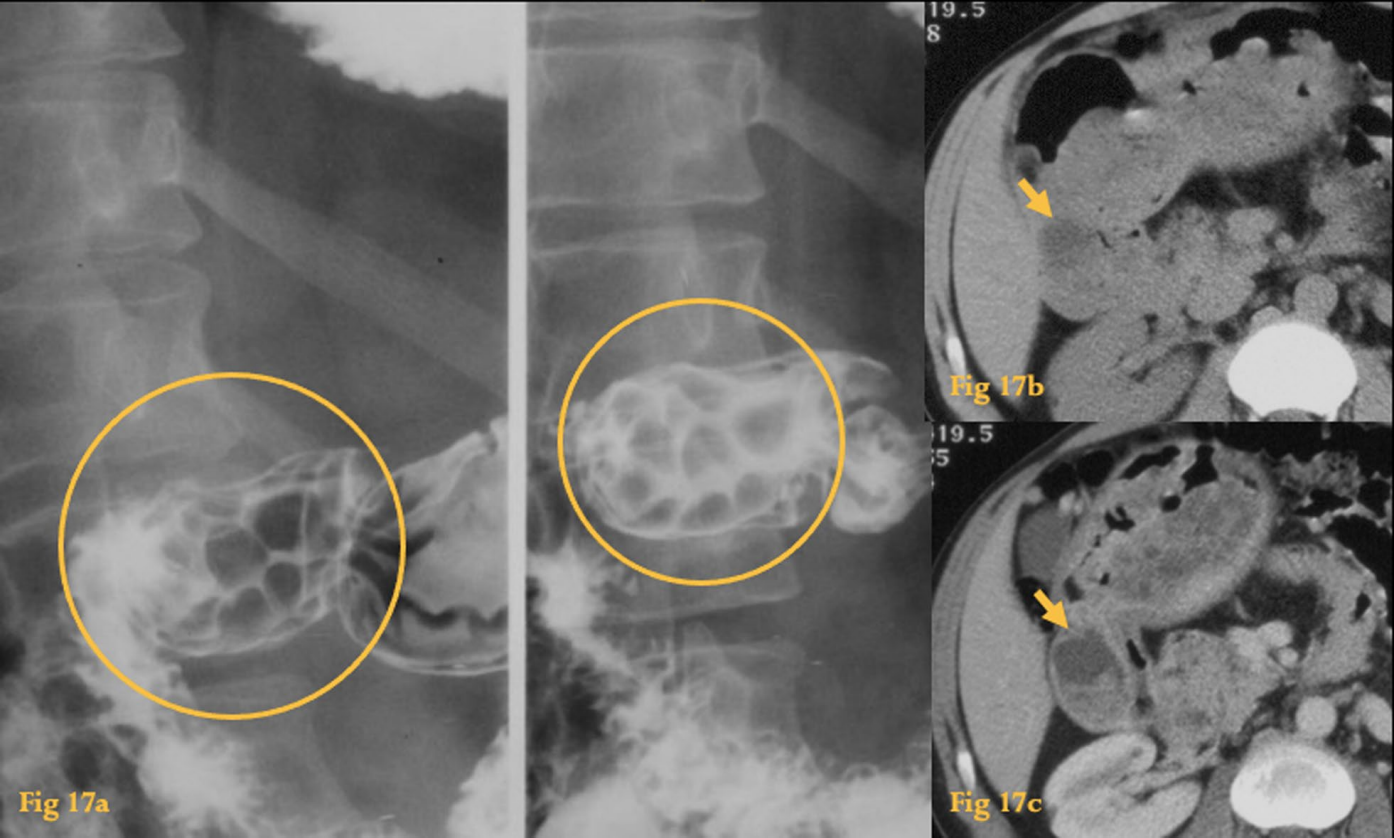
Juvenile polyposis. UGIS (a) with multiple filling defects at the duodenal bulb (circles). Unenhanced CT of another patient (b) shows a fluid density endoluminal lesion at the duodenal bulb (arrow). Enhanced CT of the same patient (c) shows several polyps at the duodenum and stomach. The biggest is located in the duodenal bulb and presents central fluid density with very little enhancement (arrow)
